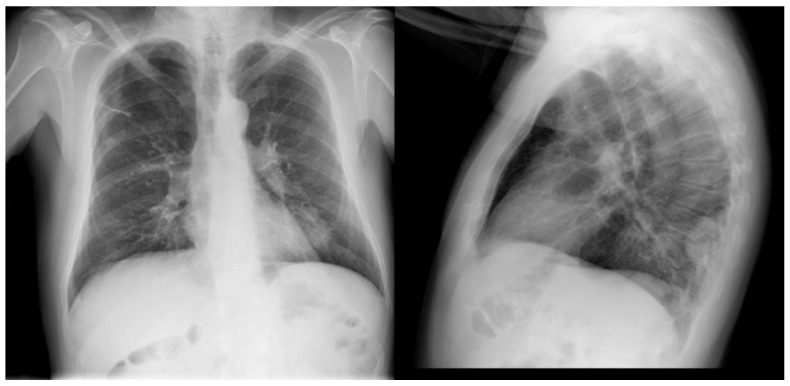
Chest X-ray in posteroanterior and lateral projection. In the left lower lobe there is a pulmonary mass of approximately 5 cm, suggestive of tumor origin, to be completed with scheduled CT study. Diminution of the vascular network with increased lucidity of the pulmonary parenchyma in both upper lobes, predominantly right, probably related to emphysematous changes. Laminar atelectasis in the LLB. Bilateral hilar prominence.
